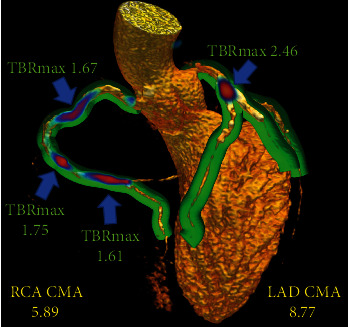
3-dimensional rendering of coronary CT angiography with superimposed tubular whole-vessel volumes of interest (light green) employed for evaluation of ^18^F-sodium fluoride uptake (blue and red). Despite the relatively lower tissue-to-background ratio maximum (TBRmax) due to multiple foci of increased ^18^F-NaF activity, the coronary microcalcification activity (CMA) in the right coronary artery (RCA) is only moderately lower than that in the left anterior descending (LAD) coronary artery which presented with a very high TBRmax. Reprinted by permission from Springer Nature: EJNMMI (Kwiecinski et al. [61]).
